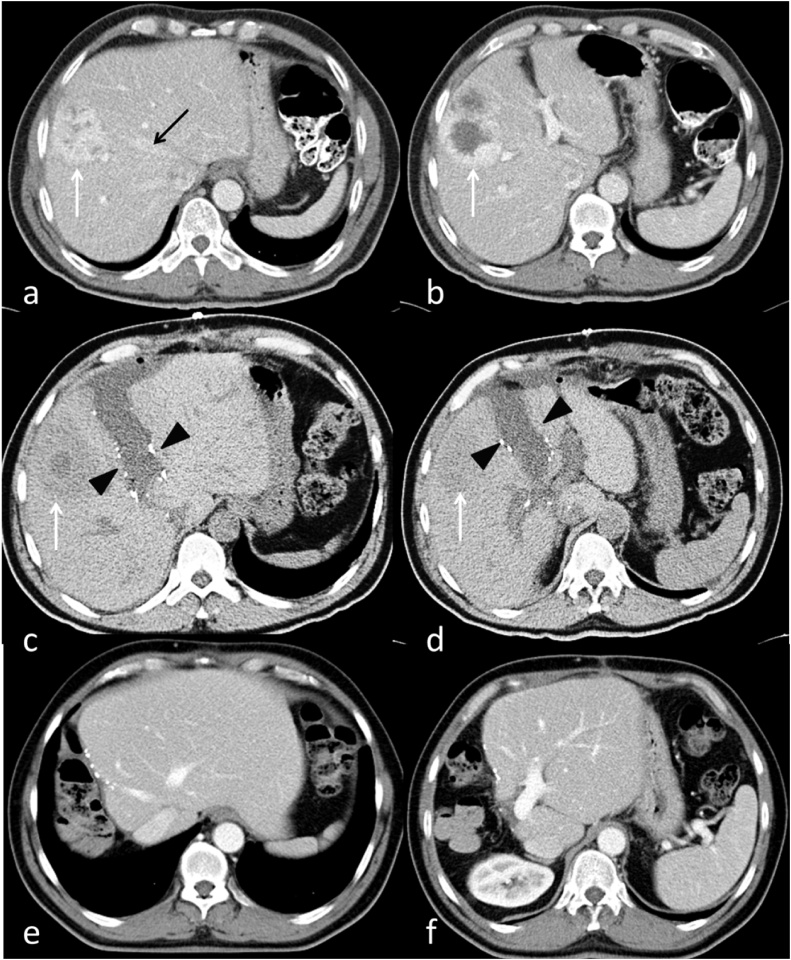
Patient 1: Preoperative contrast-enhanced CT (a,b) shows liver metastasis of the right lobe of the liver (white arrows), well encroaching on the plane of the middle hepatic vein (black arrow). Post-stage-1 unenhanced CT on postoperative day 7 (c,d) shows the liver partition plane metallic clips and postoperative fluid (black arrowheads) with normal liver parenchyma separating it from the lesion (white arrows). Post-stage-2 contrast-enhanced CT at 4 months post stage 2 (e,f) shows obvious increase in size of the remaining liver.
